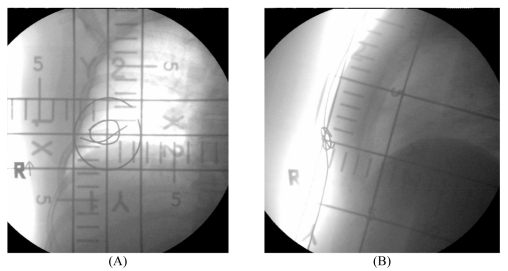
Orthogonal radiographic film of (A) Treated direction, (B) 90 degree from treated direction.
